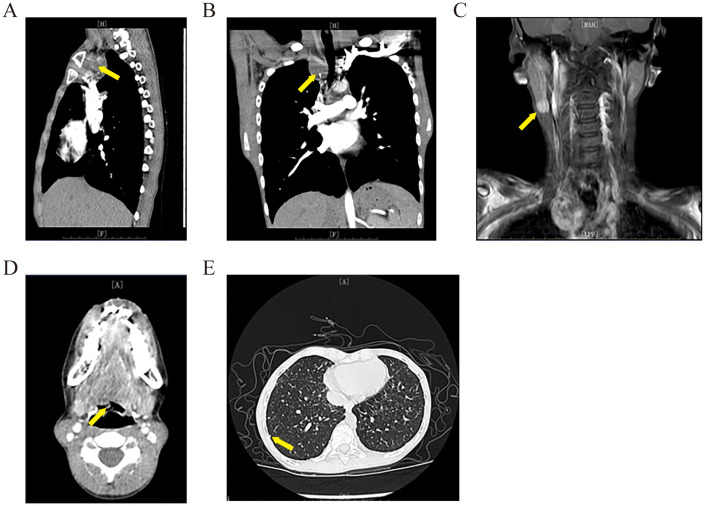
Clinical presentation of patient 1. (A) Sagittal CT image demonstrating mediastinal metastasis of medullary thyroid carcinoma in a pediatric patient following surgery; (B) Coronal CT image demonstrating mediastinal metastasis of medullary thyroid carcinoma in a pediatric patient following post-surgery; (C) Coronal MRI image demonstrating mediastinal and cervical lymph node metastasis of medullary thyroid carcinoma in a pediatric patient following surgery. (D) CT image showing a pharyngeal mass in a pediatric patient following surgery for medullary thyroid carcinoma. (E) CT image showing multiple pulmonary metastasis of medullary thyroid carcinoma in a pediatric patient following surgery.
